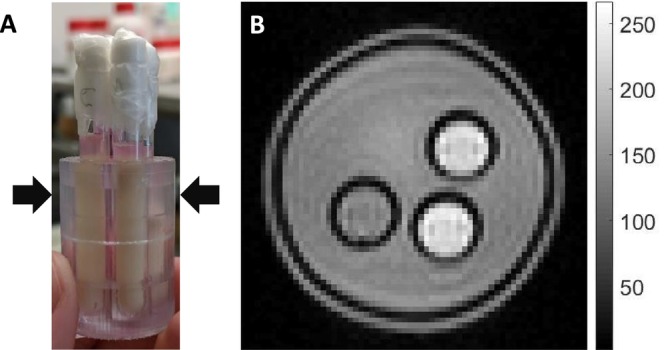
Custom 3D‐printed four‐sample holder used for MRI shown in *A*, where the arrows indicate the MRI axial image slice. The samples were control/untreated, apoptotic/cisplatin, media, and empty. A non–diffusion weighted image is shown in *B*, where the samples are blank, apoptotic, control, and media, starting at the top left going clockwise.
